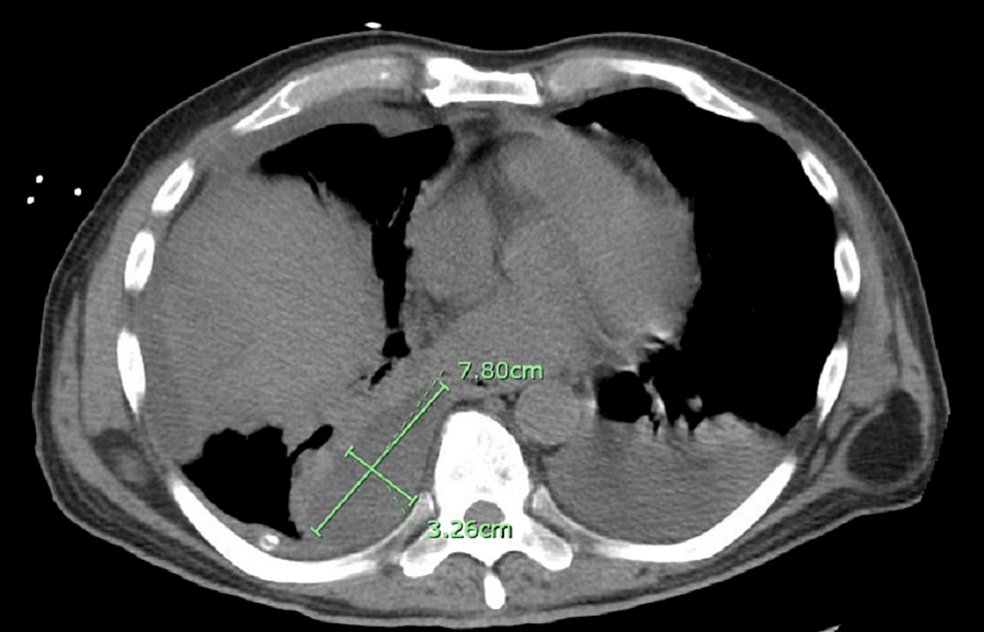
CT chest without contrast, axial cuts, showed right lung parenchyma with medial right lower loculated pleural effusion measures approximately 7.8 x 3.3 cm.
